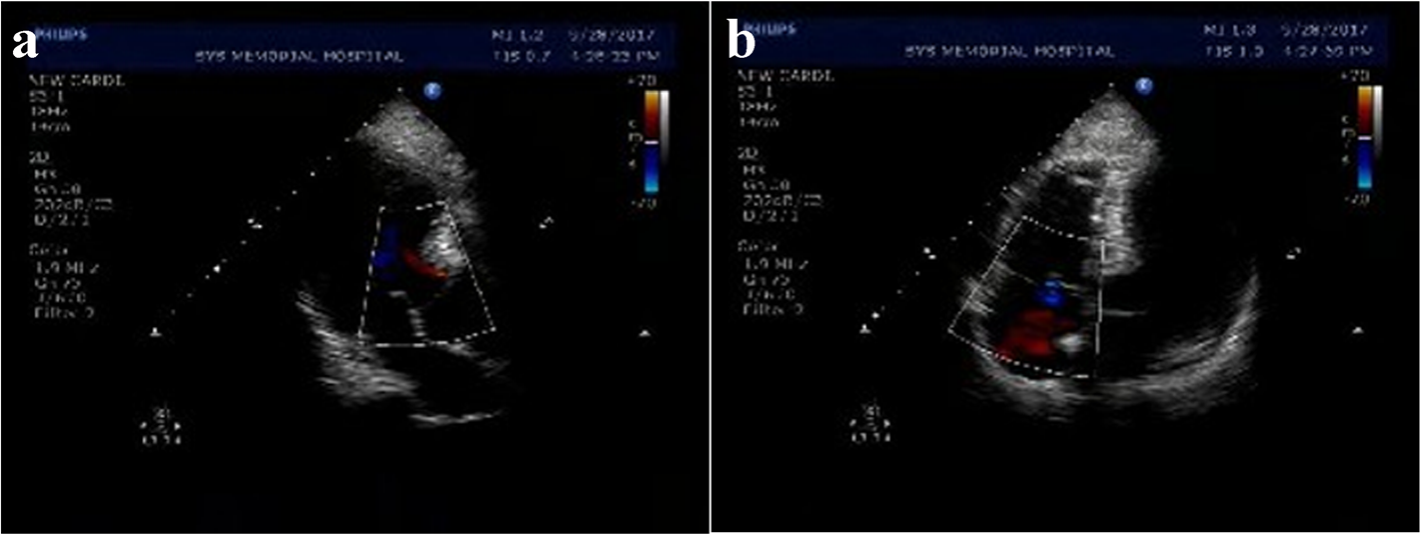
On the first day after paradoxical embolism event, the color doppler echocardiography revealed that there was no patent foramen ovale existence (a) or intracardiac defect except that the superior ventricular septum had a mild hypertrophy (15 mm) (b)
